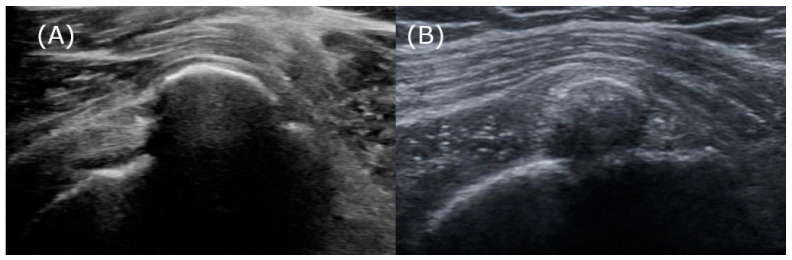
The resorptive phase calcifications, which exhibit arc-shaped attenuation and acoustic shadows beneath the bursal wall. (A) A 49-year-old female patient demonstrates calcification with pronounced arc-shaped attenuation and clear acoustic shadows. (B) A 40-year-old female patient exhibits arc-shaped attenuation with partial ultrasound penetration, allowing limited observation of the inner structure’s upper portion. The acoustic shadow is partially faint, providing a faint view of the humeral cortex.
